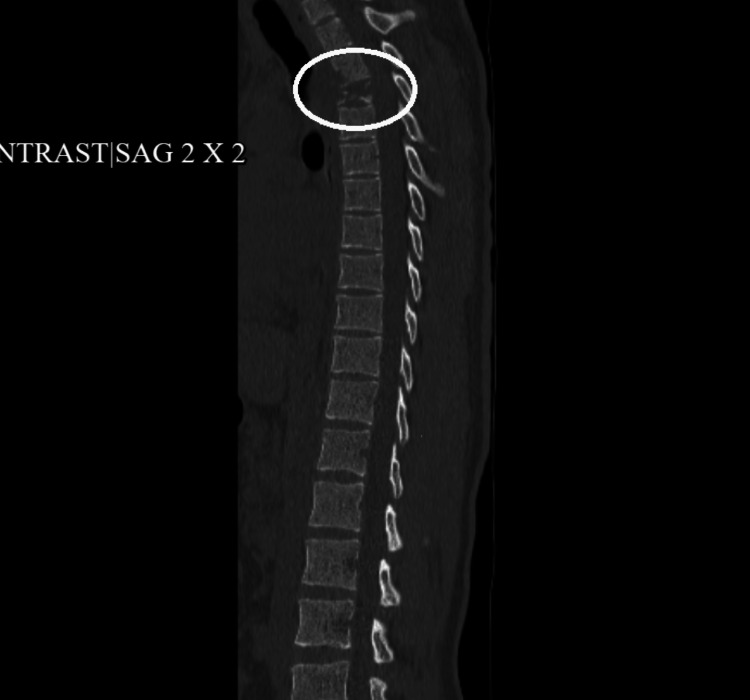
CT scan of the thoracic spine showing destruction of the T3 vertebra CT: computed tomography.
